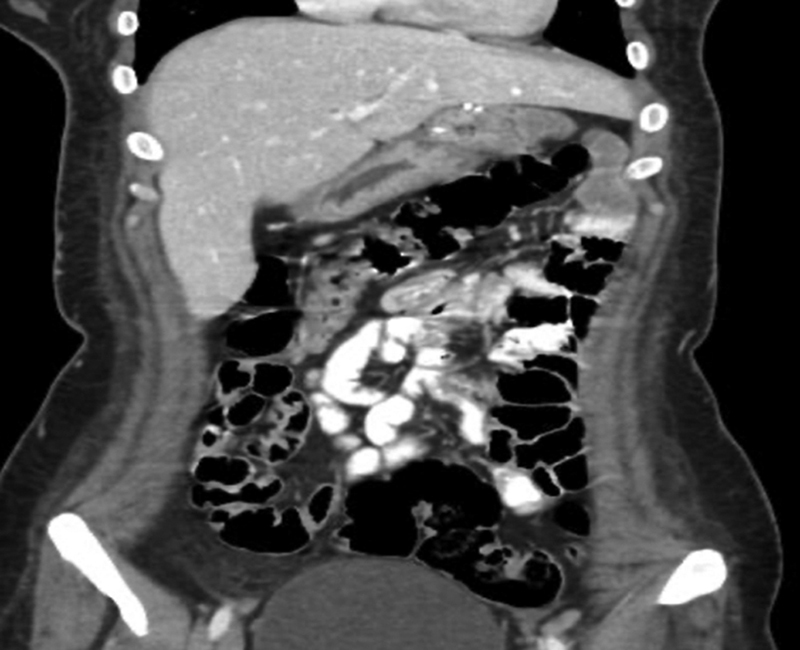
Computed tomography (CT) picture demonstrating swirling of mesentery and distended excluded stomach (case 1).
